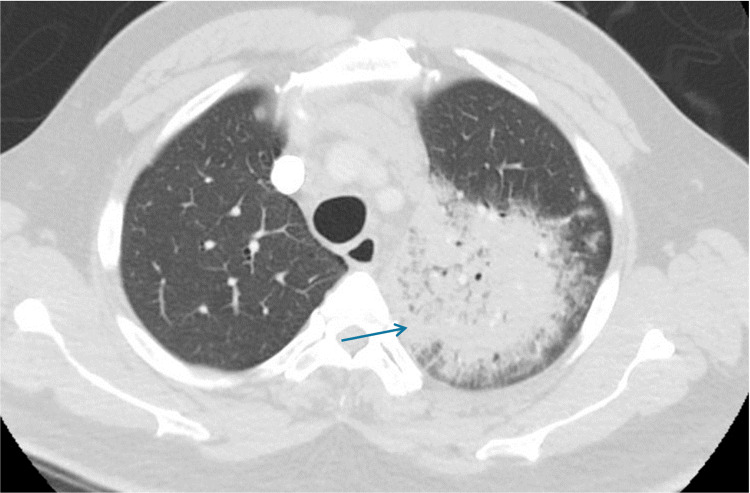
Second CT scan on the day of admission Increased mass-like consolidation left upper lobe (LUL) measuring 9.5 cm.
